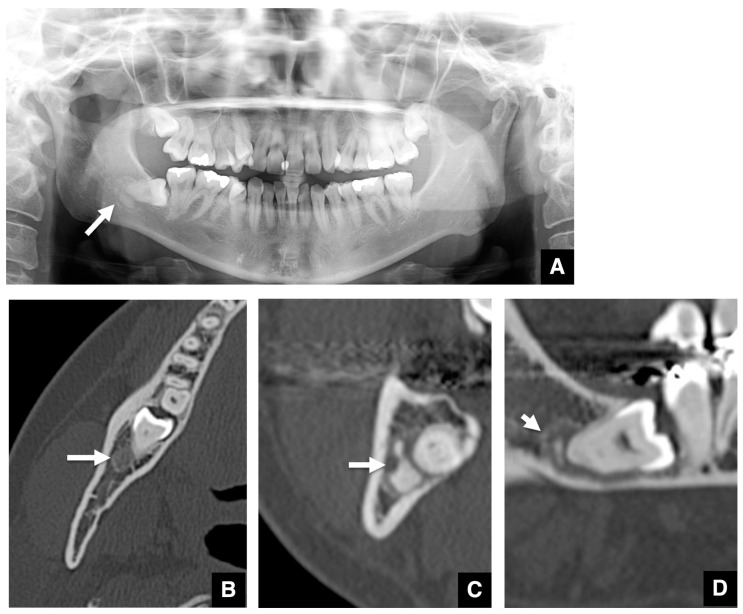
Representative panoramic radiograph and CT images of typical COD related to an embedded mandibular third molar. (A) The COD related to an embedded mandibular third molar is visualized as a round area (arrow) with a radiolucent area in the periapical region on the panoramic radiograph. A radiopaque structure within the radiolucent area is visualized. (B) The COD related to an embedded mandibular third molar is visualized as a round area (arrow) with low density in the periapical region of the tooth on axial MDCT. The mass with low density includes high-density structures. (C) The mass (arrow) and internal high-density structures are shown on coronal MDCT. (D) The mass (arrow) and internal high-density structures are shown on sagittal MDCT.
